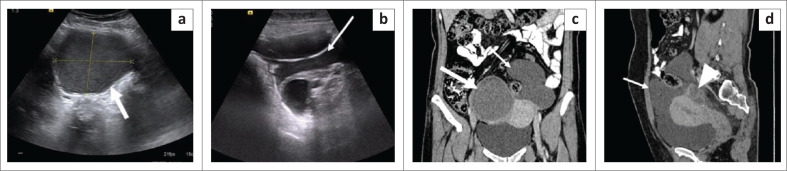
Benign cystic mesothelioma. Transverse grey scale ultrasound images (a, b) of the abdomen in a 38-year-old female reveal a well-defined cystic lesion around the right ovary with internal low-level echoes and a ground glass appearance suggestive of an endometrioma (white thick arrow in a). Another ill-defined thin-walled multiloculated cystic lesion (white thin arrow in b) is seen in midline and left side of lower abdomen and pelvis encasing the left ovary in its posterior aspect. Coronal CT image (c) of the abdomen reveals a well-defined hyperdense cyst of mean attenuation ~ 33 HU in the right adnexa (thick white arrow in c). Multilobulated fluid attenuation (~18 HU) cystic lesion with thin imperceptible walls is noticed in left lower abdomen and pelvis (thin white arrow). Sagittal reformatted CECT image (d) reveals a thin-walled multilobulated cystic lesion anterior and superior to uterus, encasing the left ovary posteriorly (arrowhead). Minimal fluid is observed within the endometrial cavity. No calcification or mural nodule is noted within the lesion.
